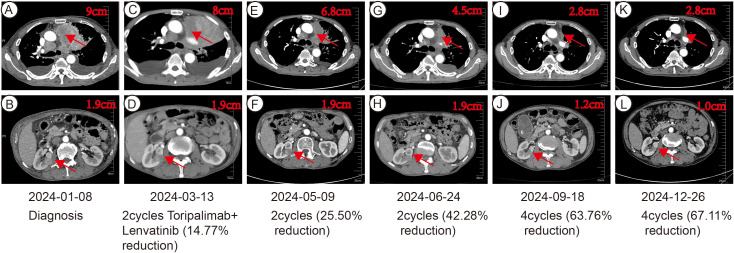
CT imaging assessment of left lung lesion and right kidney mass throughout the course of disease corresponding to their respective time points. (A, B) CT image at initial diagnosis on January 8, 2024. (C, D) CT image on March 13, 2024. (E, F) CT image on May 9, 2024. (G, H) CT image on June 24, 2024. (I, J) CT image on September 18, 2024. (K, L) CT image on December 26, 2024.
